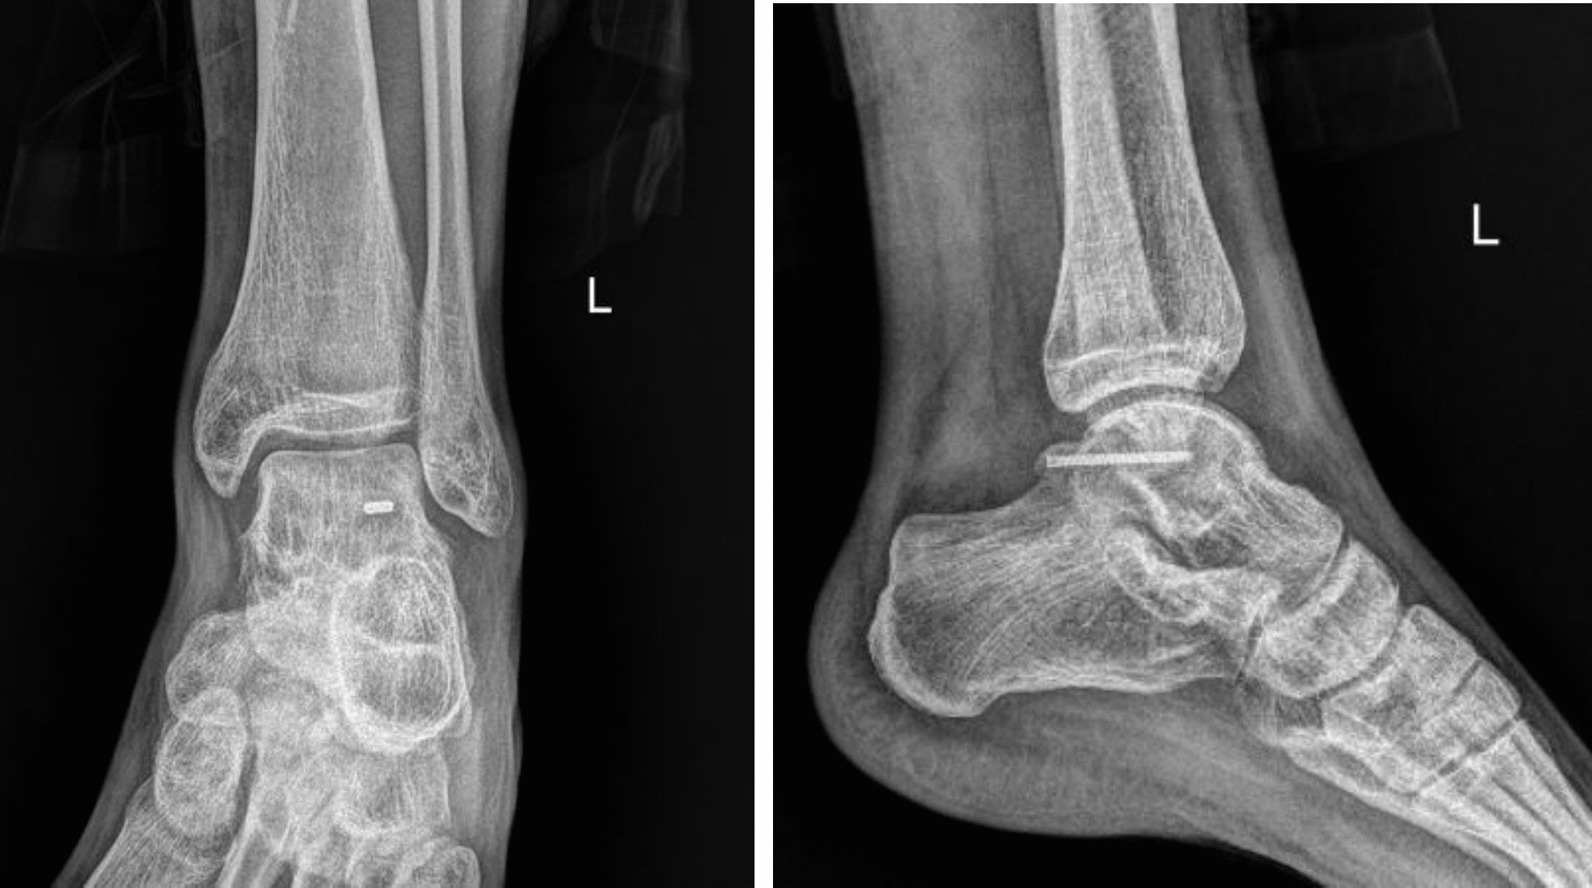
X-ray 6 weeks after surgery (AP, lateral)
